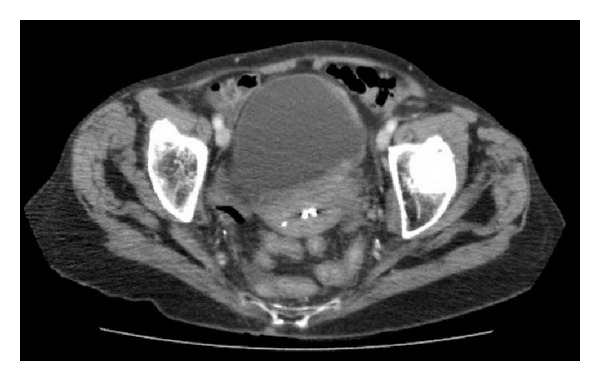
Abdominal and Pelvic CT showing a thickened posterior and left lateral wall.
